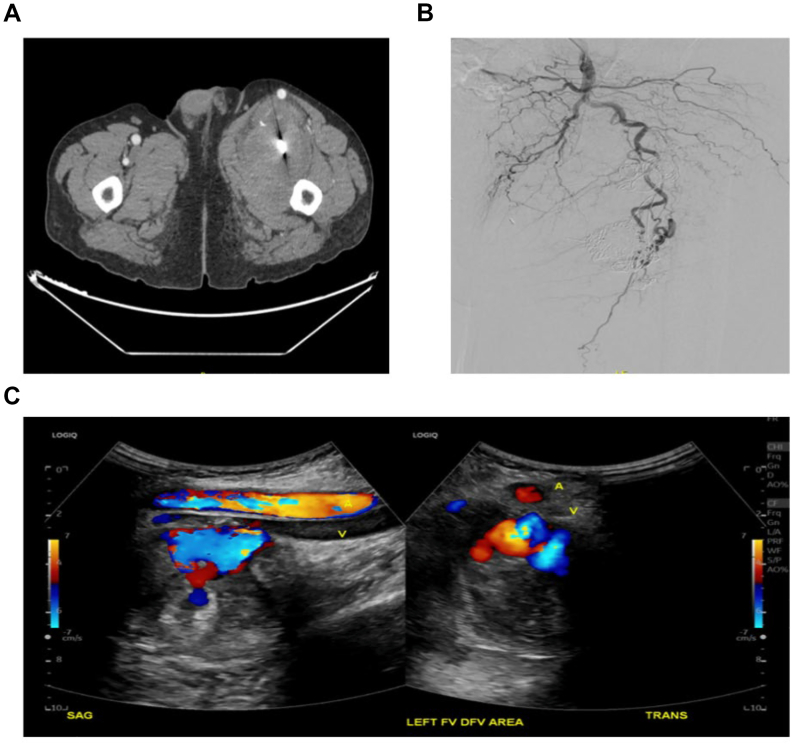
(A) Computed tomography angiography (CTA) of left thigh with left profunda femoris aneurysm with significant size increase but no filling. (B) Conventional angiogram that showed no flow into the sac of the aneurysm. (C) Duplex ultrasound examination showing internal residual flow in the inferior portion of the bilobed aneurysm.
